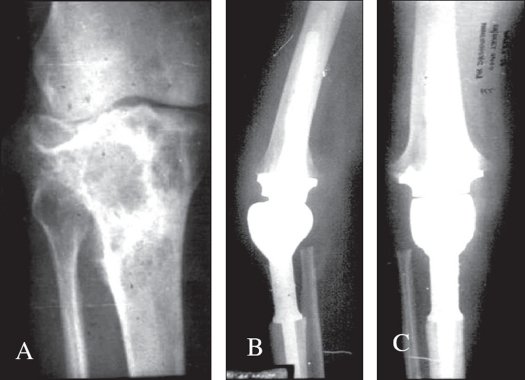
A) X-ray knee (A.P.) of a patient with GCT of proximal tibia. B), C) Ten years follow-up X-ray (Lat and AP view) after wide resection and custom mega prosthetic replacement
